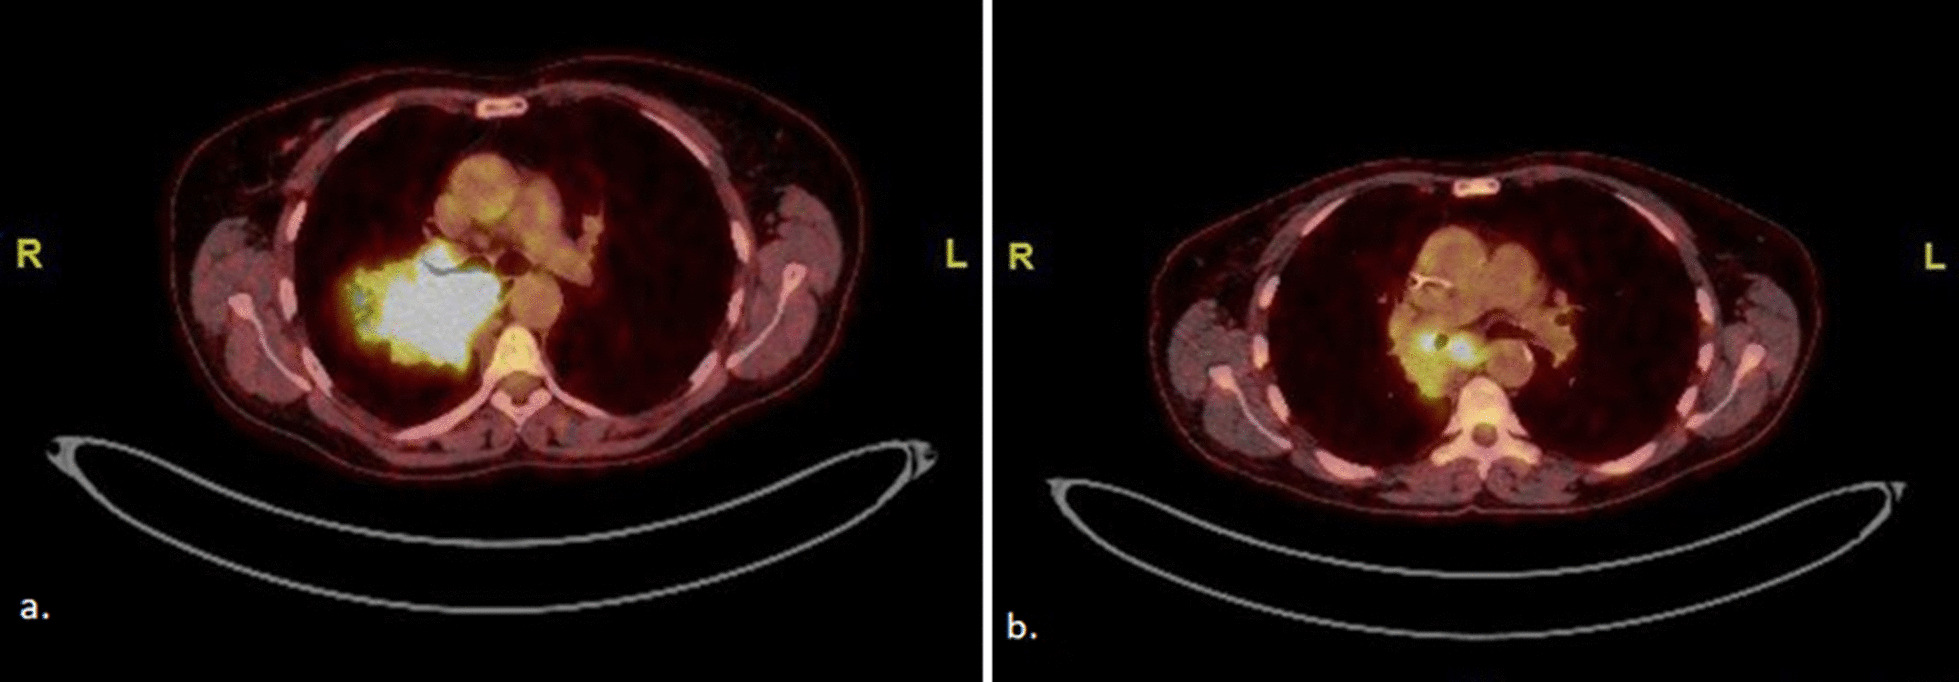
^18^F-FDG PET/CT. a Hypermetabolic parahilar primary lung lesion (SUV_max_ 23.9). b Partial morphometabolic response (SUV_max_ 8.1) of the primary lesion following four cycles of carboplatin-pemetrexed and pembrolizumab as first-line treatment
